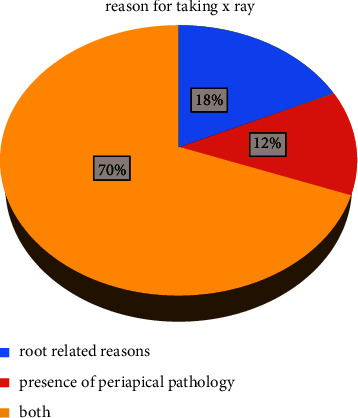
Reasons for taking X-ray.
